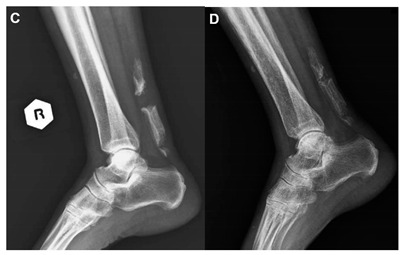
Akhaddar, 2014 ^c^ [7]	A 52-year-old man. This image submission included plain radiographs with a lateral and an antero-posterior view of the right leg and ankle. Arrows indicate a 10-cm ossification of the Achilles Tendon without a fracture (Images E and F). The patient presented after acute trauma to the foot, and the ossified mass was an incidental finding. The patient had no symptoms of the lower leg at presentation and had no history of metabolic or systemic illness nor ankle trauma. Surgery was not performed due to the lack of symptoms.
